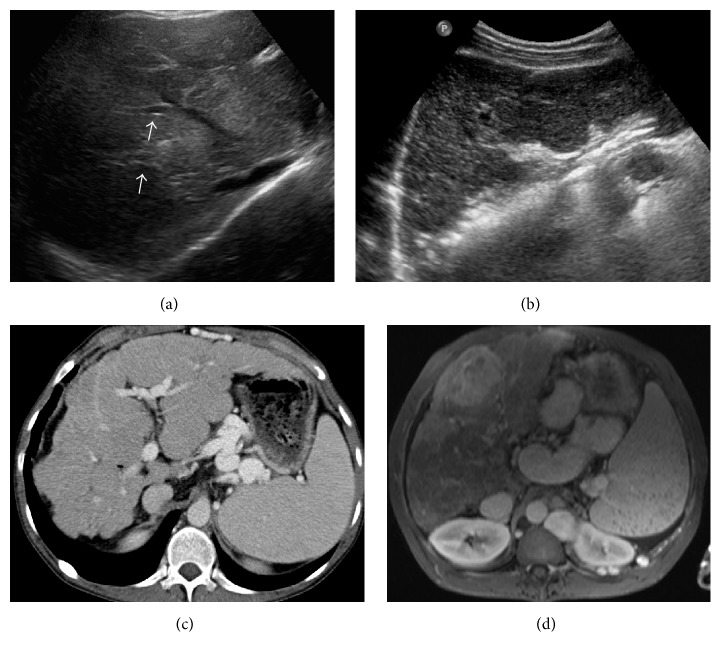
(a) Longitudinal US image of a 32-year-old male with cystic fibrosis demonstrating periportal echogenicity (arrows) consistent with periportal fibrosis. (b and c) Transverse US image and axial CT demonstrating a shrunken, nodular right lobe of liver with relative left lobe hypertrophy, varices, and splenomegaly in a CF patient with deranged LFTs at time of imaging. (d) Delayed enhanced T1-weighted axial MRI demonstrating regenerative nodules surrounded by bands of enhancing fibrotic liver parenchyma.
